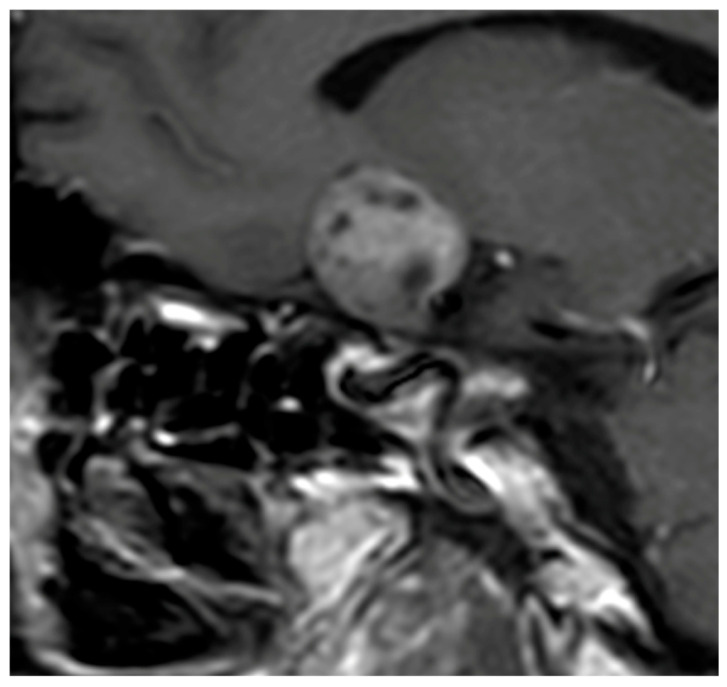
Sagittal T1-weighted image after Gadolinium injection showing a heterogeneous enhancement of the pituitary mass consistent with a NFPMA.
